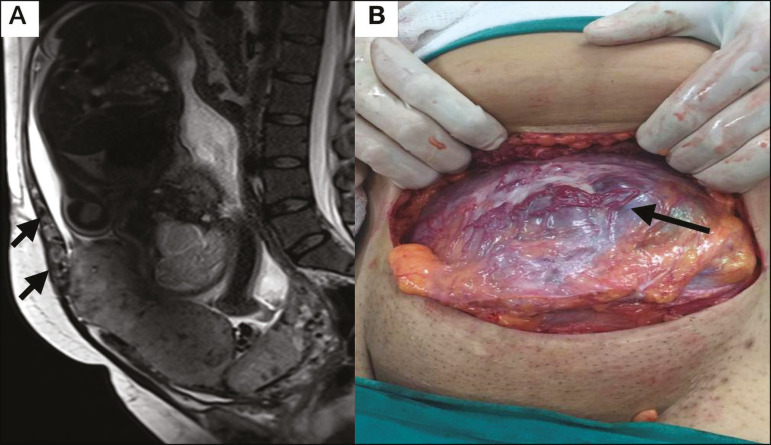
A: Sagittal T2-weighted image showing subserosal hypervascularity of the uterus with flow voids (arrows). B: Intraoperative appearance.
